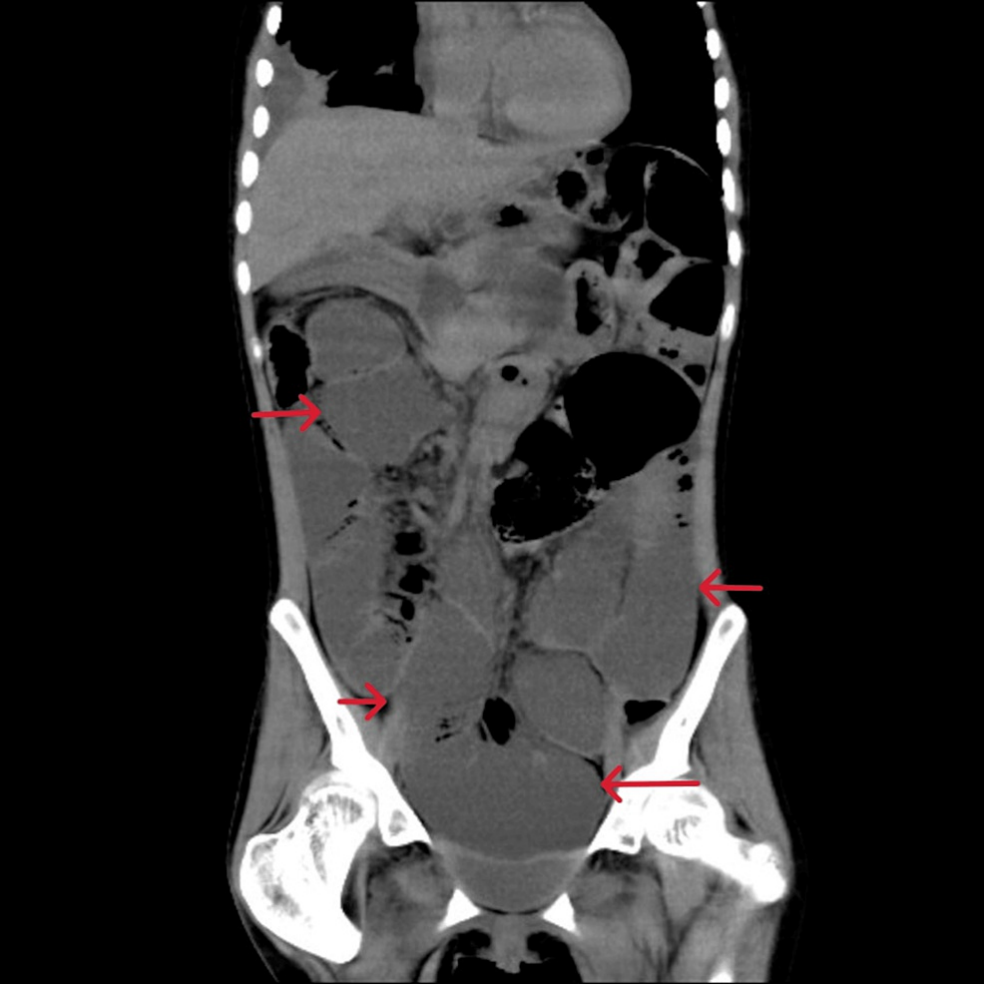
Plain CT of the abdomen (Coronal). Red arrows show dilated, fluid-filled small bowel loops.
